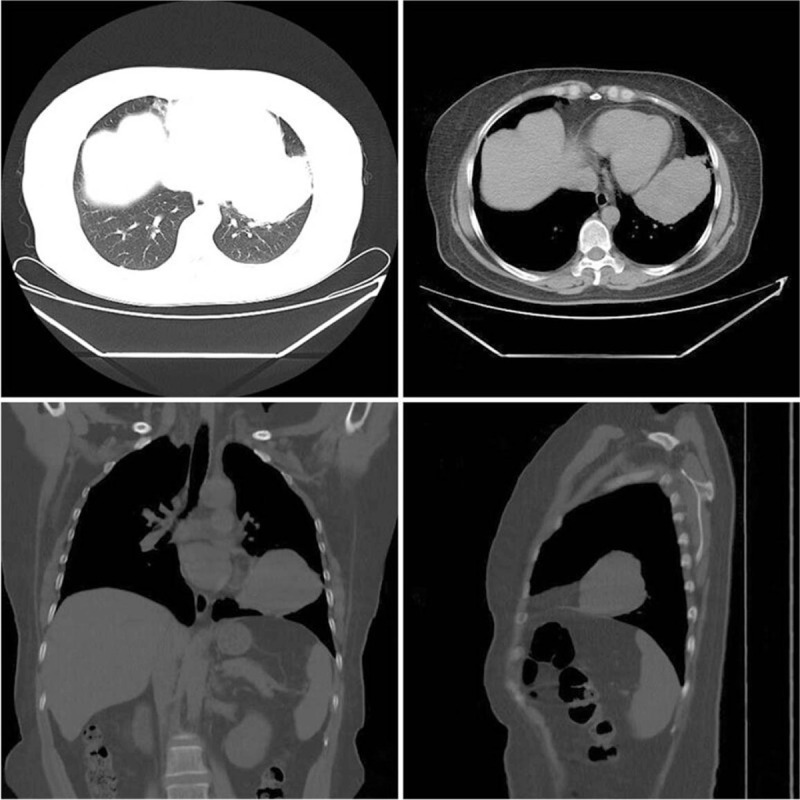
Chest computed tomography (CT) scan showing a soft tissue mass in the tongue segment of the left superior lobe of the left lung. Tumor size was approximately 74 × 51 mm, and the CT value was 33 HU. A small liquefaction component was seen in the soft tissue mass.
